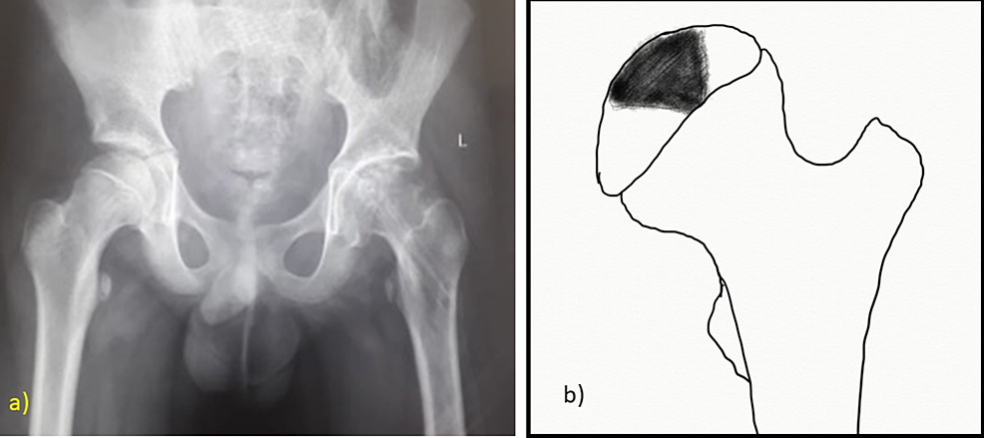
Case Example: Segmental Avascular Necrosis of Femoral Head A 12-year-old male: closed reduction and screw fixation were done. a) Radiographs one month after screw removal showing segmental involvement of the head (Ratliff type II). b) Pictorial representation of the areas of avascular necrosis of the femoral head.
